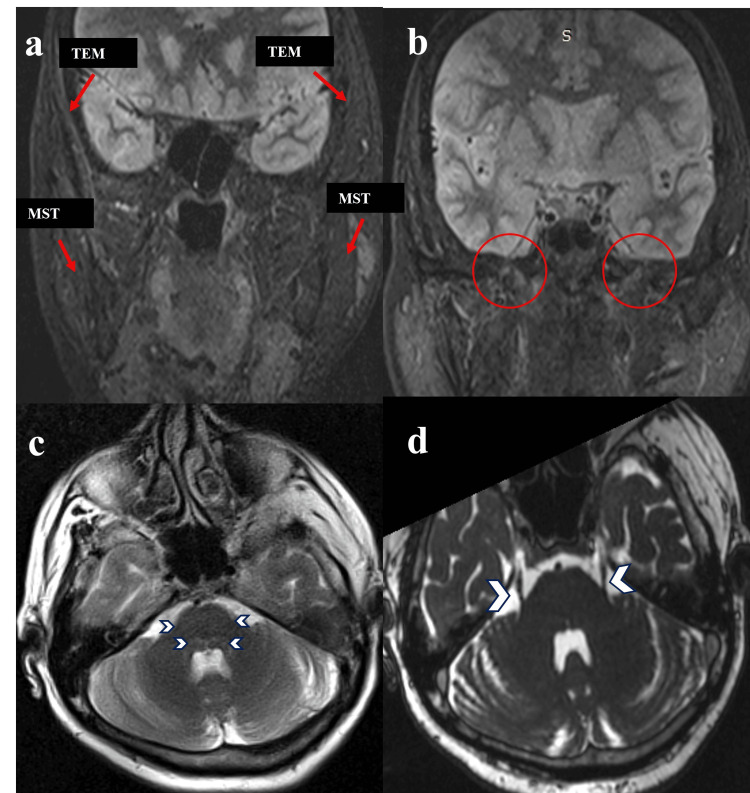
Fat-Suppressed and High-Resolution MRI Evaluation of the Trigeminal Nerve Pathway Coronal STIR images (a) demonstrate marked fatty atrophy of the left temporalis (TEM) and masseter (MST) muscles without associated muscle edema, supporting chronic denervation changes. The coronal STIR image through the skull base (b) demonstrates no abnormal soft tissue lesion or abnormal signal intensity along the expected foraminal course of the mandibular division of the trigeminal nerve bilaterally (circles). Axial T2-weighted image at the pontine level (c) demonstrates no focal signal abnormality within the pontine tegmentum. High-resolution 3D constructive interference in steady state (3D-CISS) image (d) shows symmetrical cisternal segments of both trigeminal nerves (arrowheads) without thickening, compression, or signal abnormality.
